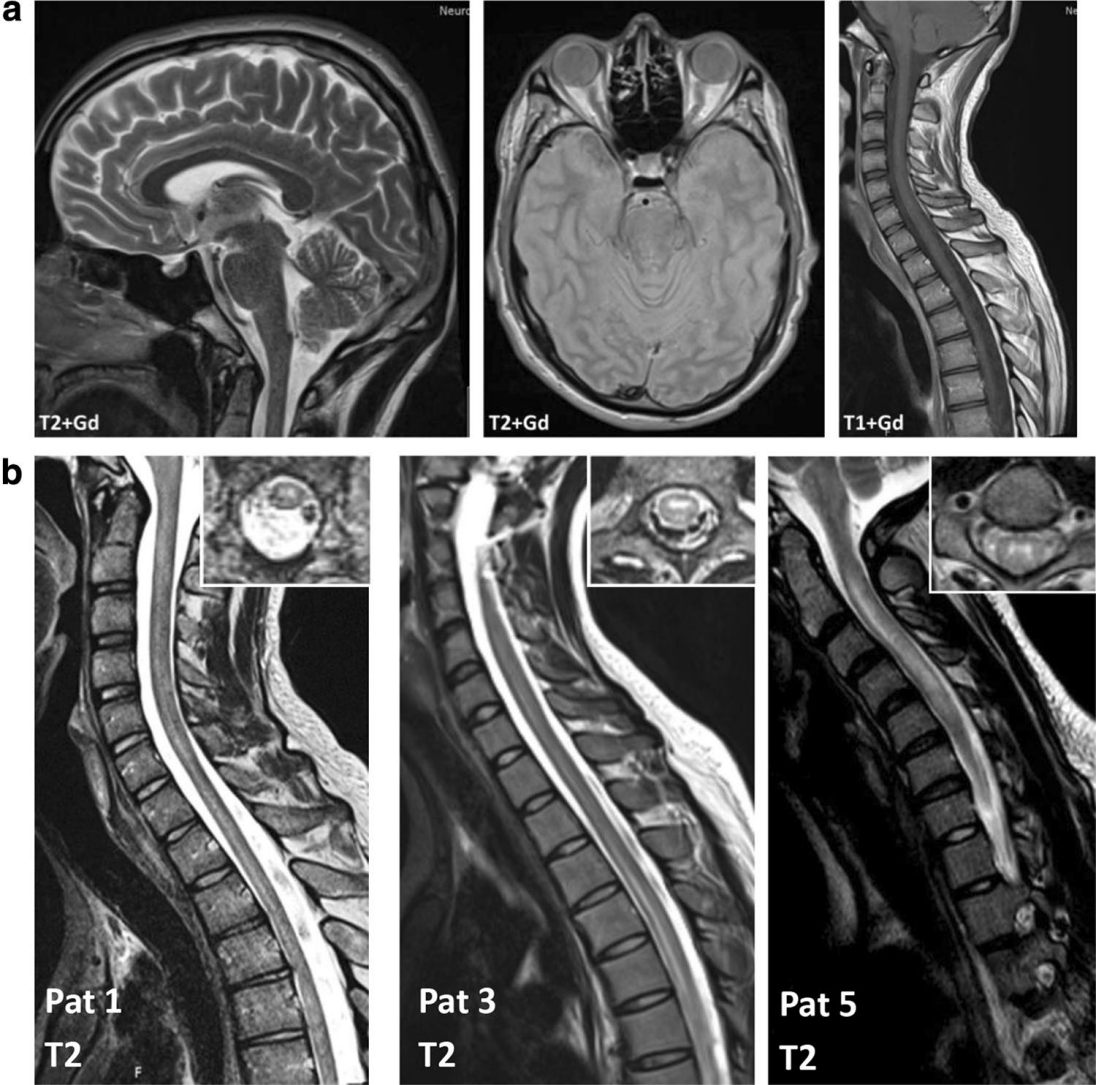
aRepresentative examples of brain MRI scans from one patient during peak of disease symptoms. No lesions were detected in either T2- or T1-weighted MRI images with gadolinium (Gd). b Representative examples of spinal cord lesions detected by MRI in each patient. Sagittal T2-weighted spinal MRI performed at disease onset show longitudinal lesions extending throughout the spinal cord. Inserts show axial sections of the spinal cord at lesion level
